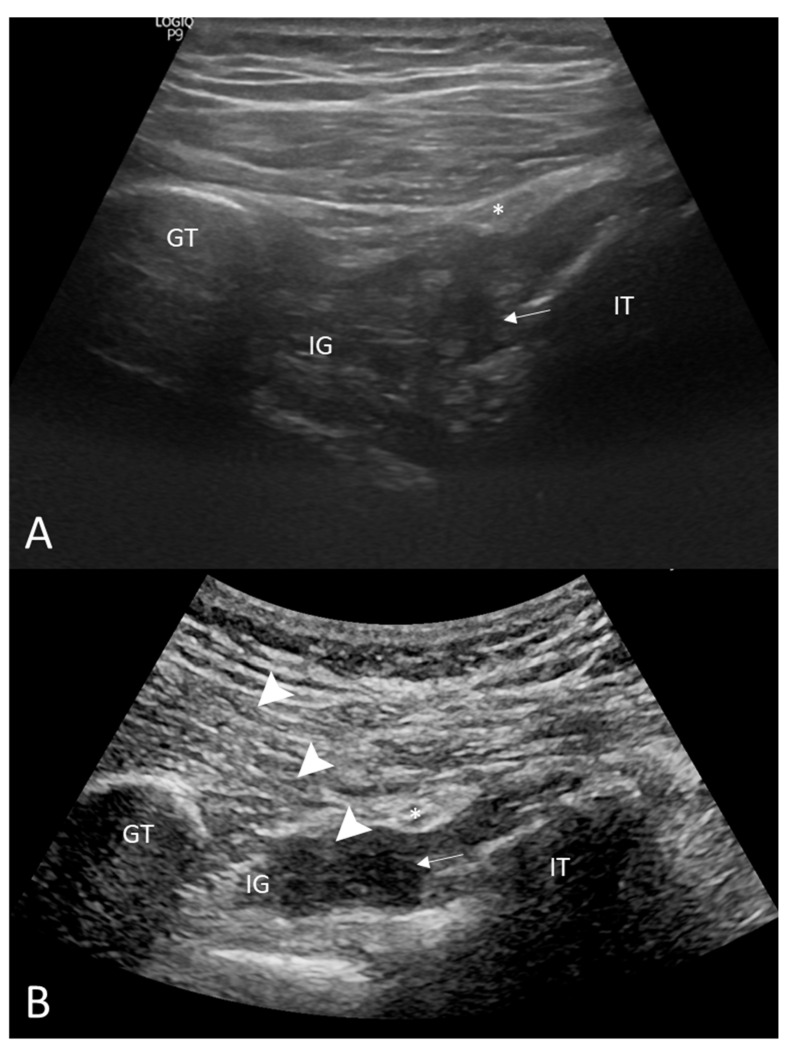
Musculoskeletal sonograph of inferior gemellus. (A) Musculoskeletal sonography showed a swollen muscle with hematoma (arrow) between the ischial tuberosity and medial surface of greater trochanter of the femur. Sciatic nerve (asterisk) was superficial to the muscle. The inferior gemellus strain with hematoma was highly suspected before an MRI scan was performed. (B) Musculoskeletal sonography showed ultrasound-guided platelet-rich plasma injection (arrowhead) to the inferior gemellus. GT = greater trochanter; IG = inferior gemellus; IT = ischial tuberosity.
